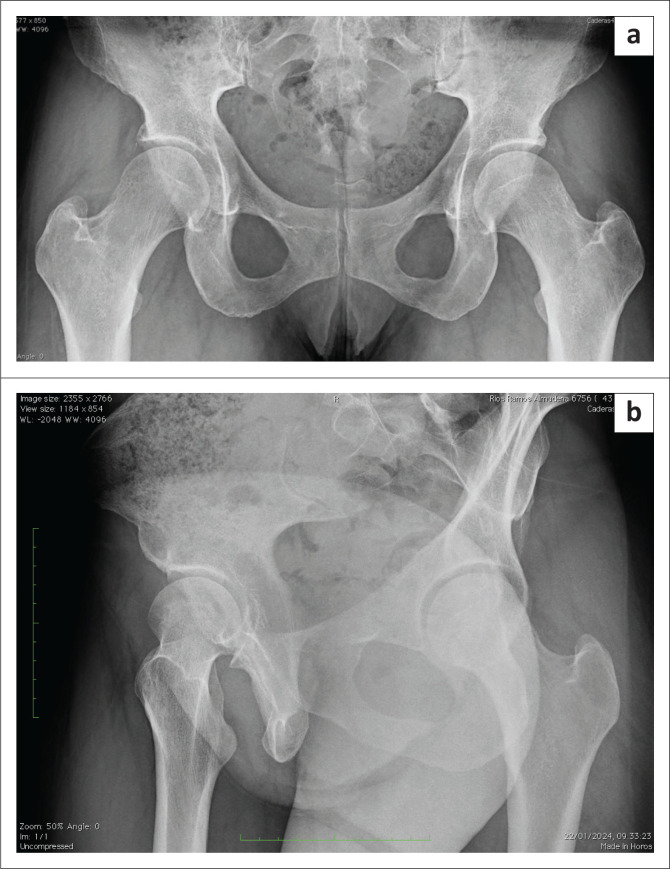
(a) Post-operative plain anteroposterior pelvis radiograph showing almost complete resolution of the calcifications at both hip joints after right hip arthroscopy with residual faint linear calcifications. (b) Right hip. Profile radiograph showing complete removal of the calcifications and cam excision.
